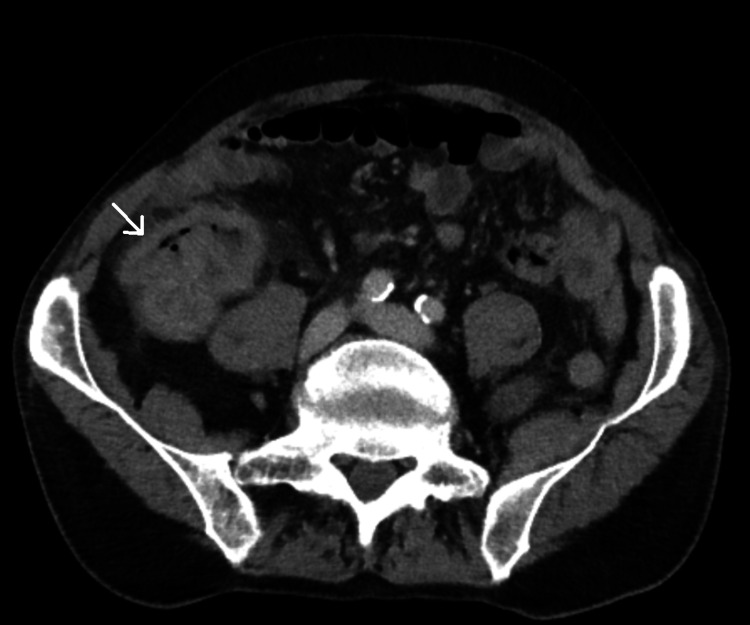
Axial CT scan of the abdomen showing the ascending colon mass. White arrow indicating the location of the ascending colon tumor. CT, computed tomography
